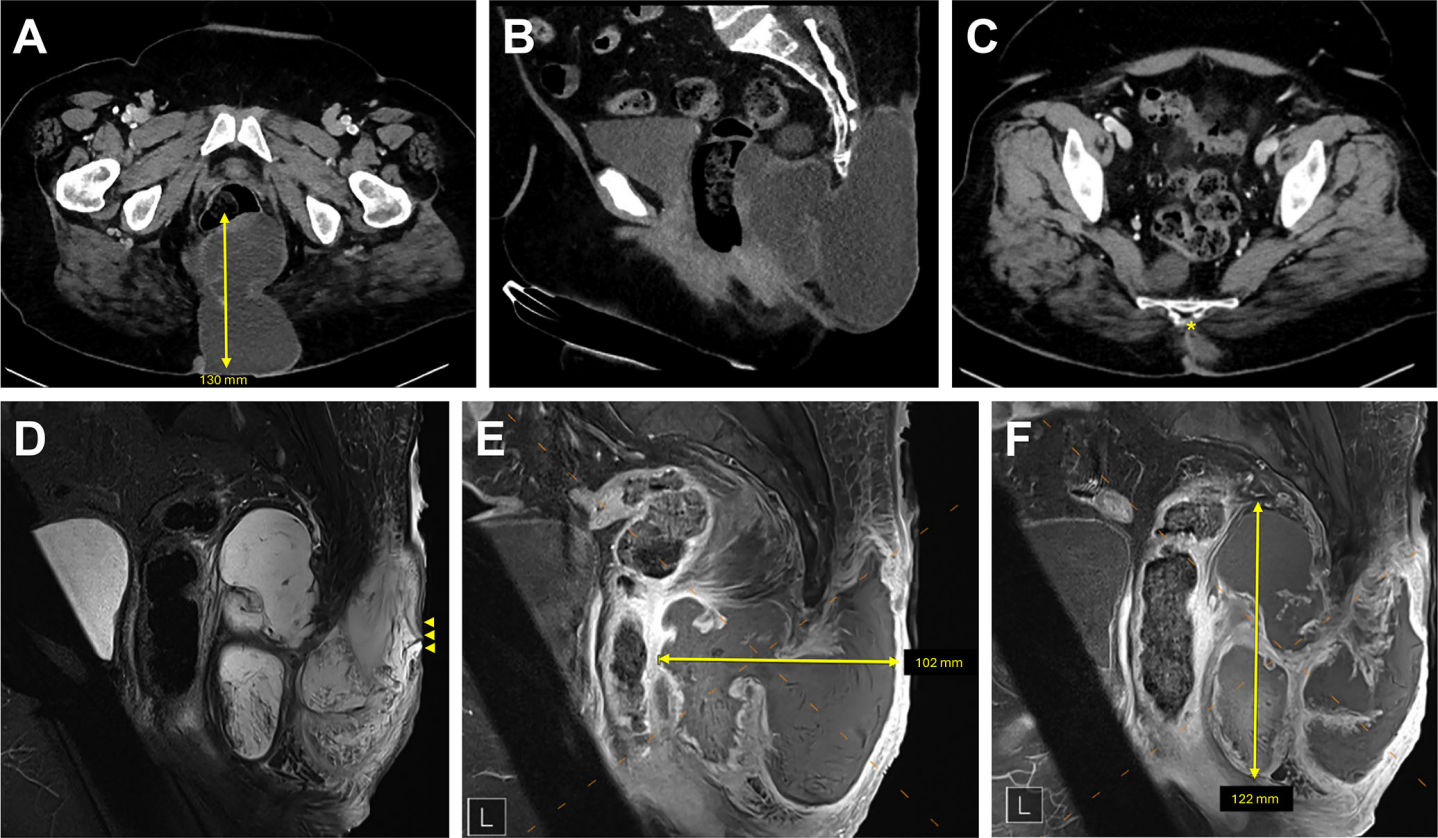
Initial abdominal and pelvic computed tomography (CT) and magnetic resonance imaging (MRI) at diagnosis. (A). Axial CT image showing the mucinous adenocarcinoma measuring 130 mm in maximal diameter (yellow arrow). (B). Sagittal CT image demonstrating extent of tumor in posterior soft tissues. (C). Axial CT image demonstrating possible erosion of the coccygeal tip (yellow star). (D). Sagittal MRI image revealing tumor-associated ulceration (yellow arrowheads). (E). Sagittal MRI image depicting tumor dimension width of 102 mm (yellow arrow). (F). Sagittal MRI image depicting tumor dimensions length of 122 mm (yellow arrow).
